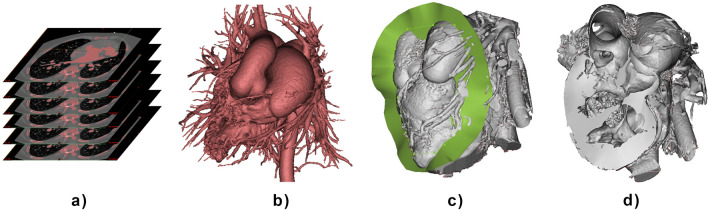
Process of segmentation and modeling. (a) Segmentation of CT images. (b) Blood pool model. (c) Cutting of the myocardial model. (d) Myocardial model cut with a flexible surface.
